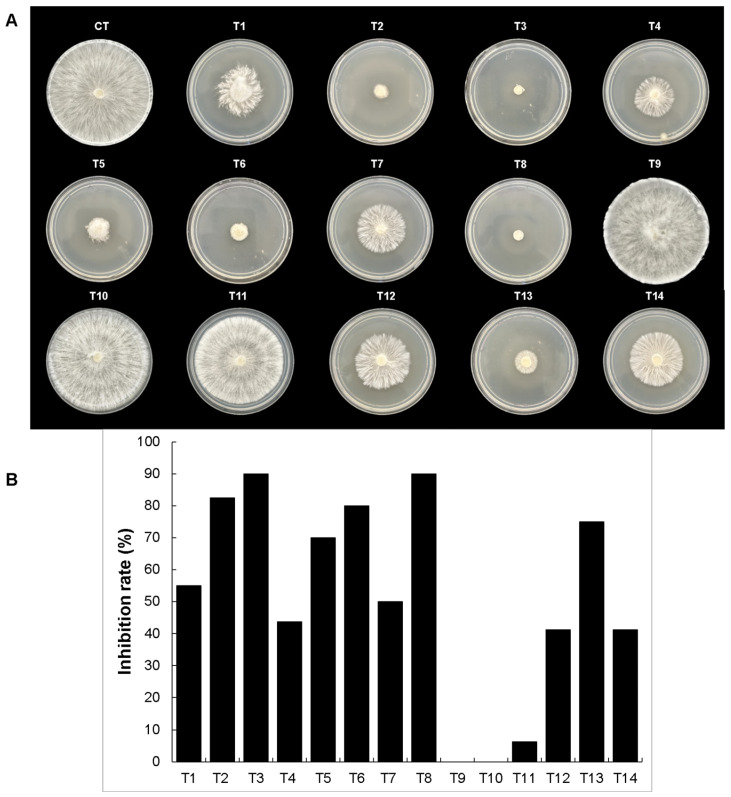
The mycelial growth inhibition rates of standard VOCs against *S. rolfsii*. The treatments applied were T1, T2, and T3 with 2,5 dimethyl pyrazine concentrations of 100 µL, 150 µL, and 200 µL; T4, T5, and T6 with 100 µL, 150 µL, and 200 µL of acetoin; T7 and T8 with 10 µL and 20 µL of benzaldehyde; and T9, T10, and T11 with 50 µL, 100 µL, and 150 µL of 2-butanone. Also, the combination of pyrazine, acetoin, and benzaldehyde was used with different concentrations. T12 used 10 µL of each compound, T13 used 15 µL of each compound, and T14 used 10 µL of 2,5 dimethyl pyrazine and 20 µL of the other compounds. (A) The radial mycelial growth inhibition effects of the VOCs used against *S. rolfsii*. (B) Percentages of mycelial inhibition with the mean value of two essays. CT mean is the control without treatment.
